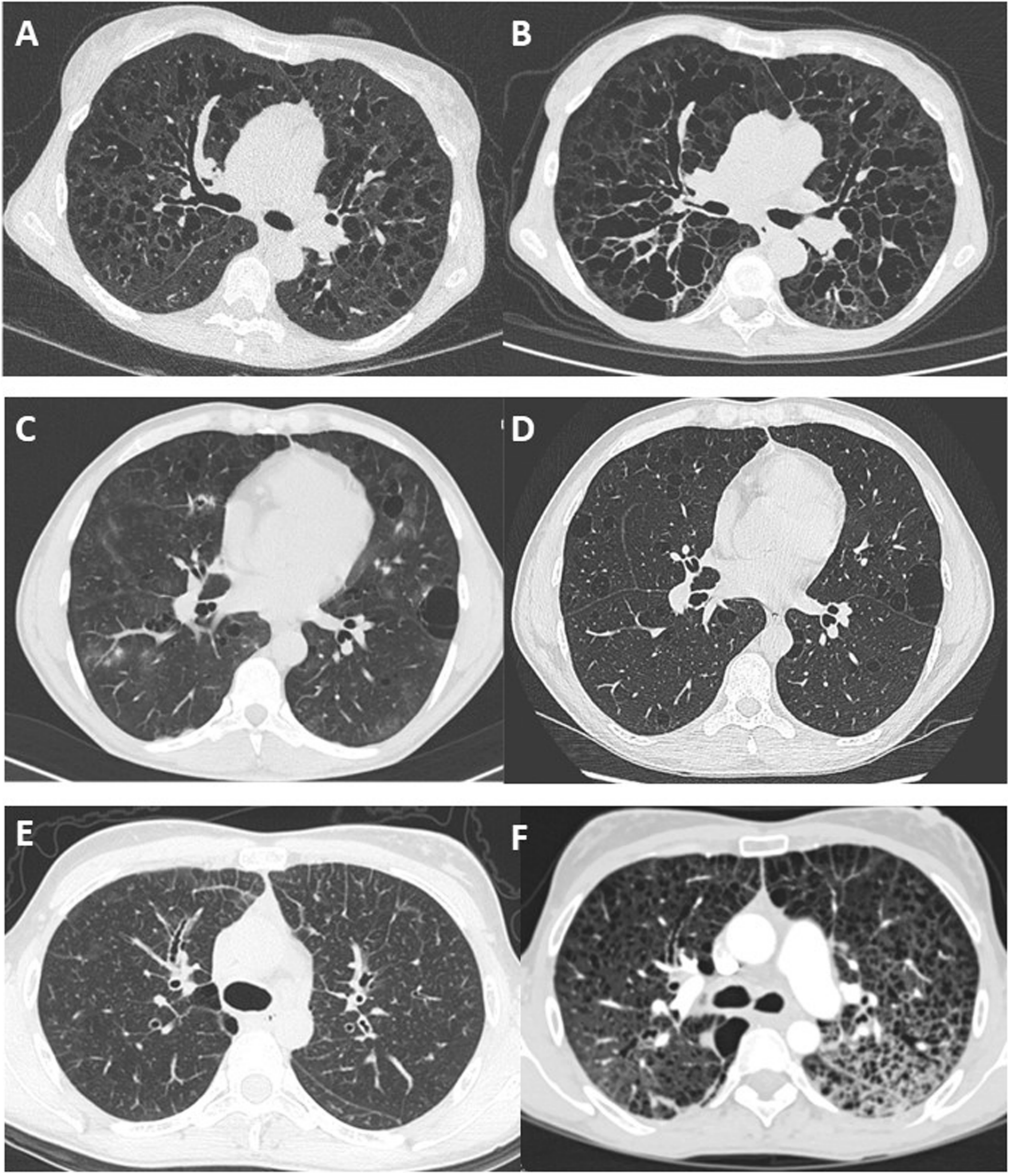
Long-term change in chest CT features in 3 patients showing 3 patterns. A and B CT scan at baseline and after 8 years in a patient with diffuse cystic bronchiectasis. C and D CT scan at baseline and after 1 year in a patient with regular round cysts of various sizes. E and F CT scan at baseline in a patient with micronodules and interlobular reticulation, and 7 years later with multiple cysts and ground glass attenuation
